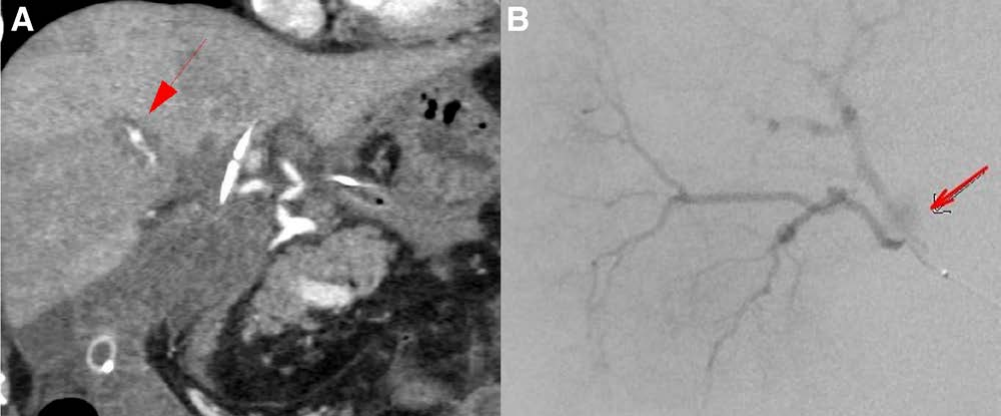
Biliary bleeding was observed following biliary reconstruction surgery. (A) Prompting a contrast-enhanced CT scan which revealed an intrahepatic aneurysm(➡). (B) Emergency IVR was performed. Angiography revealed a ruptured aneurysm in the A8 hepatic artery (➡), which was successfully coiled for hemostasis 2 years ago. CT = computed tomography, IVR = interventionl radiology.
